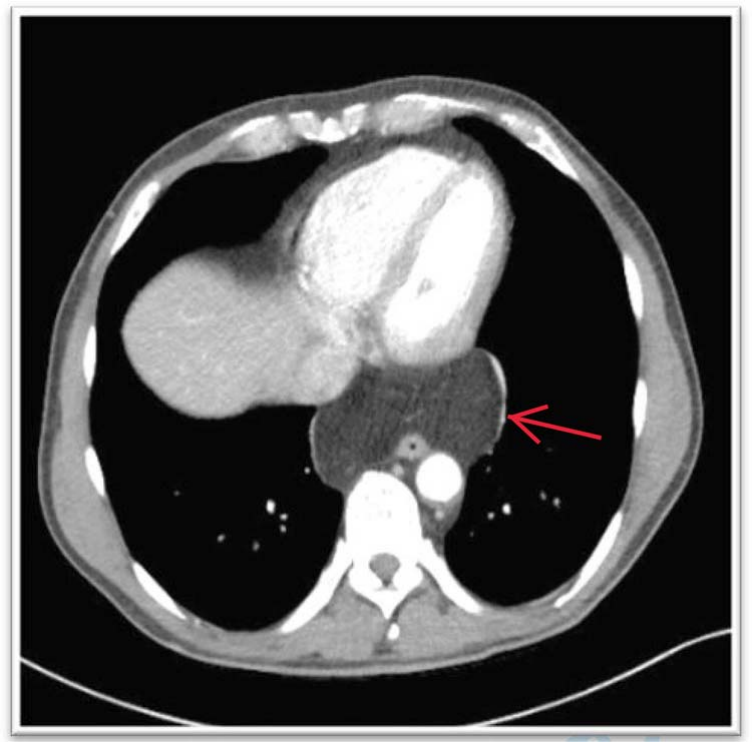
CT showing hypodense mass in the lower posterior mediastinum.
